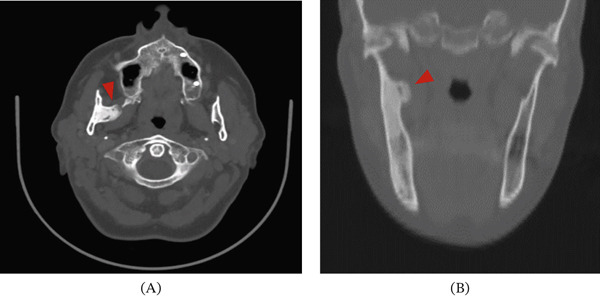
(A) Axial and (B) coronal CT images showing a mushroom‐like bone lesion with lobulated margins arising from the lingual surface of the right mandibular ramus. The lesion is located anterior to the mandibular foramen (arrowhead). The lesion extends up to the external pterygoid lamina and between the internal and external pterygoid muscles into the right masticator space.
